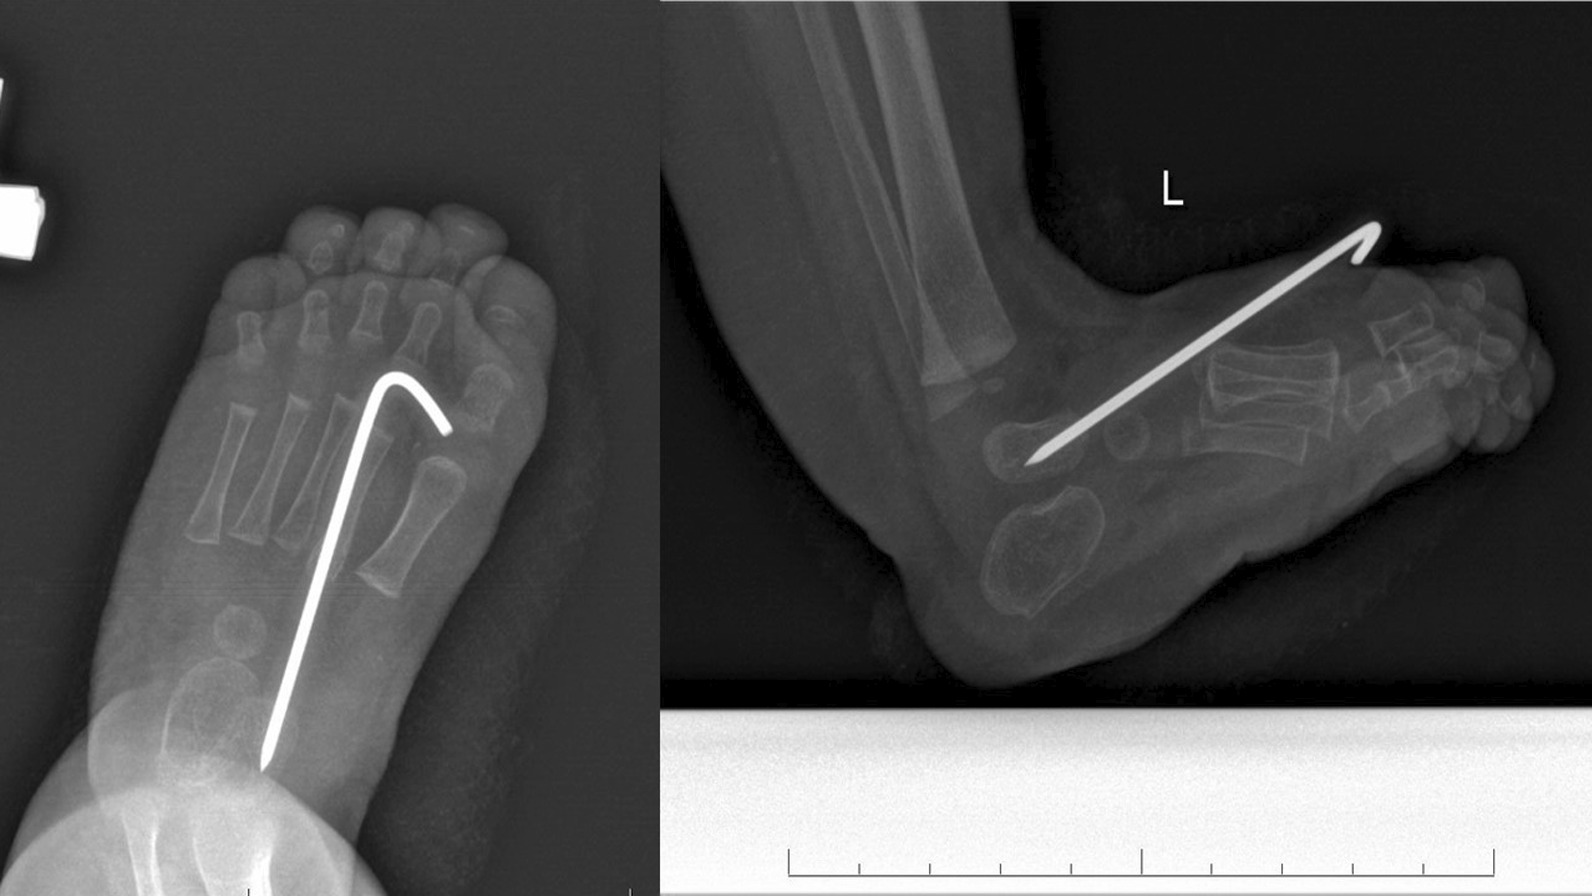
Case 1 AP and L X-ray after surgery
